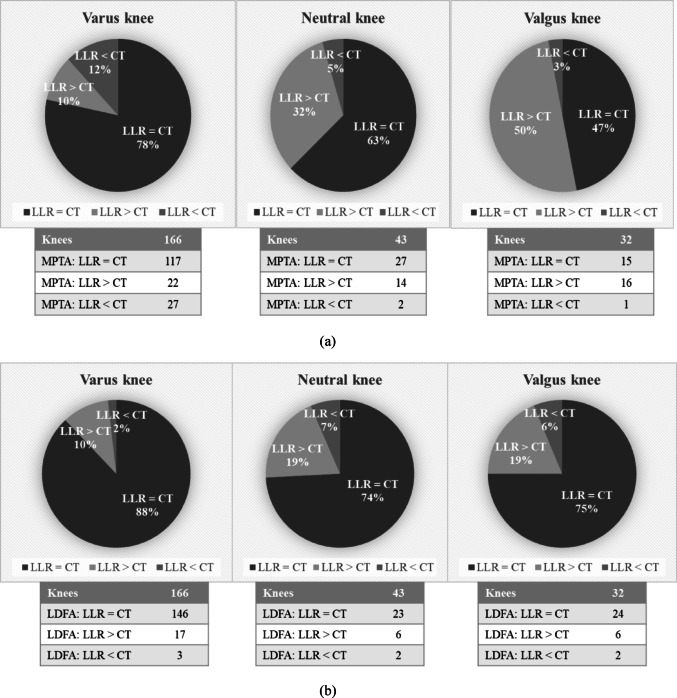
Association between preoperative coronal knee alignment and discrepancies between long-leg radiograph (LLR) and computed tomography (CT) measurements of medial proximal tibial angle (MPTA) and lateral distal femoral angle (LDFA). Varus knees were defined as preoperative mHKA > 3° varus, neutral knees as − 3° to + 3°, and valgus knees as > 3° valgus. Discrepancy categories were defined as agreement within 2° (LLR = CT), LLR > CT (> 2°), and LLR < CT (> 2°)
